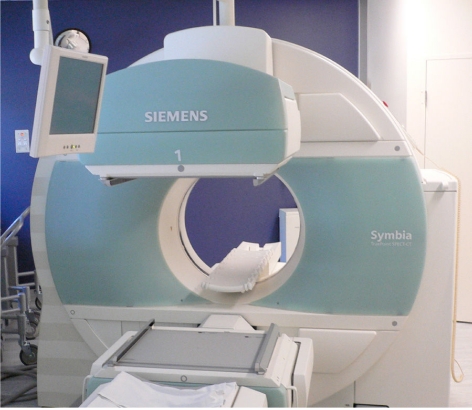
Modern hybrid SPECT-CT scanners incorporate multi-detector CT. This is the Siemens Symbia 6 system installed in these authors’ facility.
